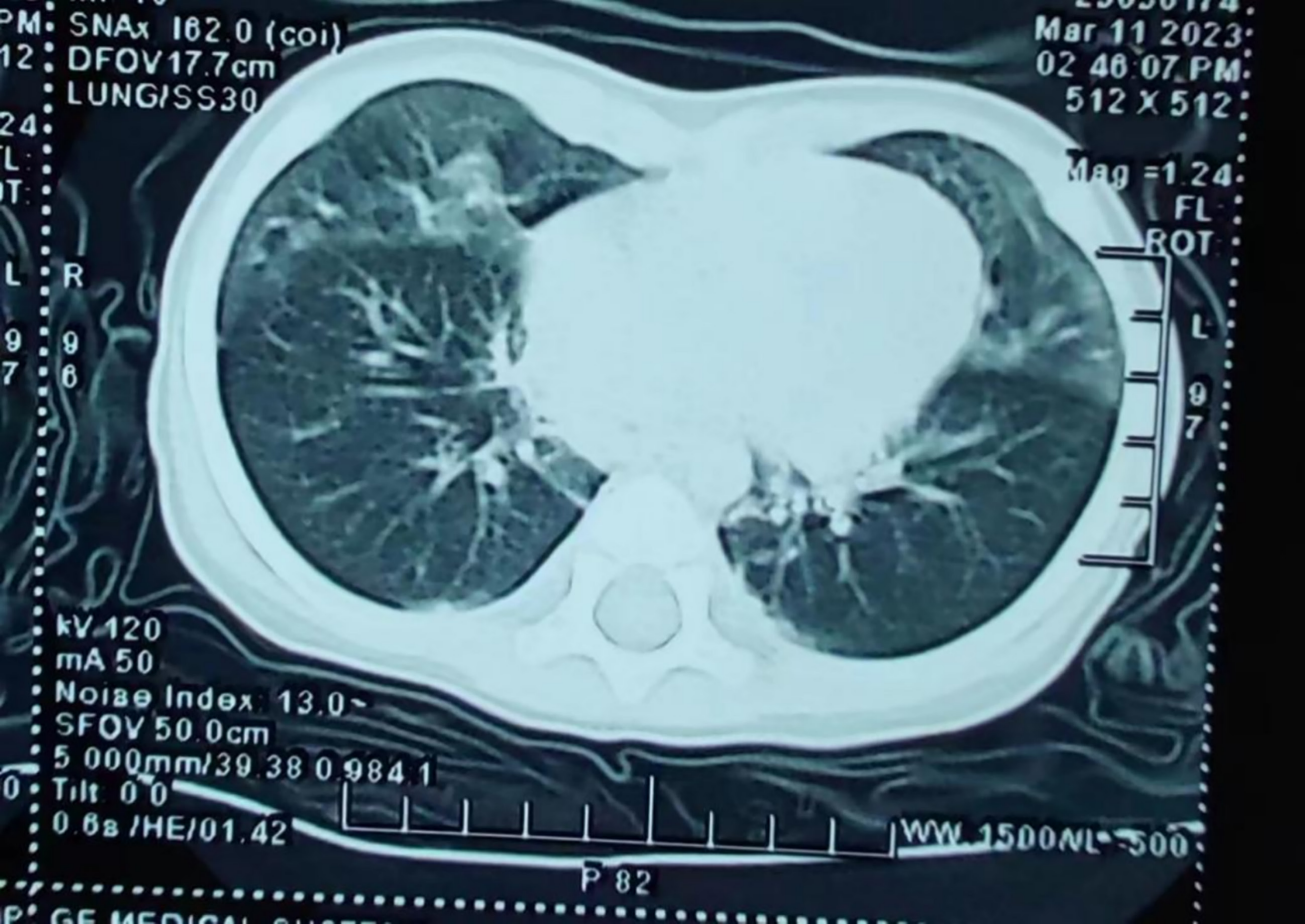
Typical ground glass opacities and air retention on chest CT of children with NEHI.
